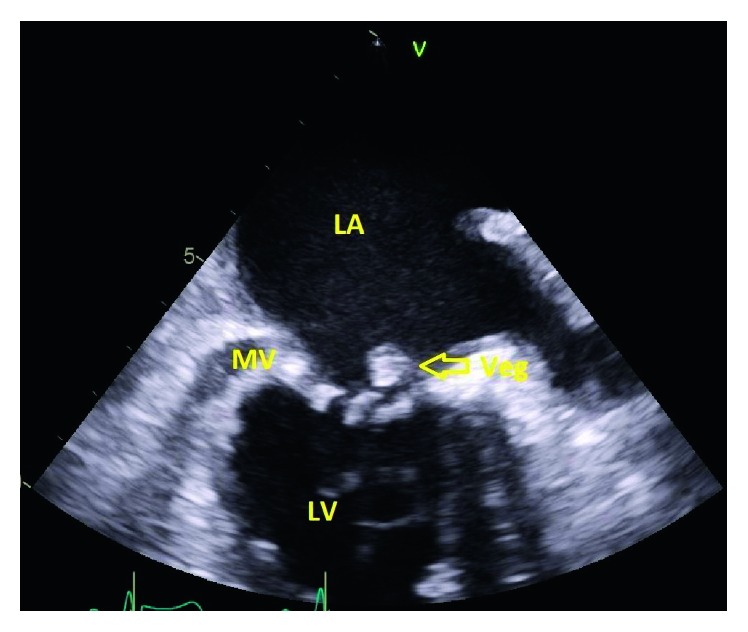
Transesophageal echocardiogram (TEE) showing a 0.63 cm × 0.54 cm vegetation on the posterior aspect of the mitral leaflet (indicated by arrow). LA: left atrium; MV: mitral valve; LV: left ventricle.
